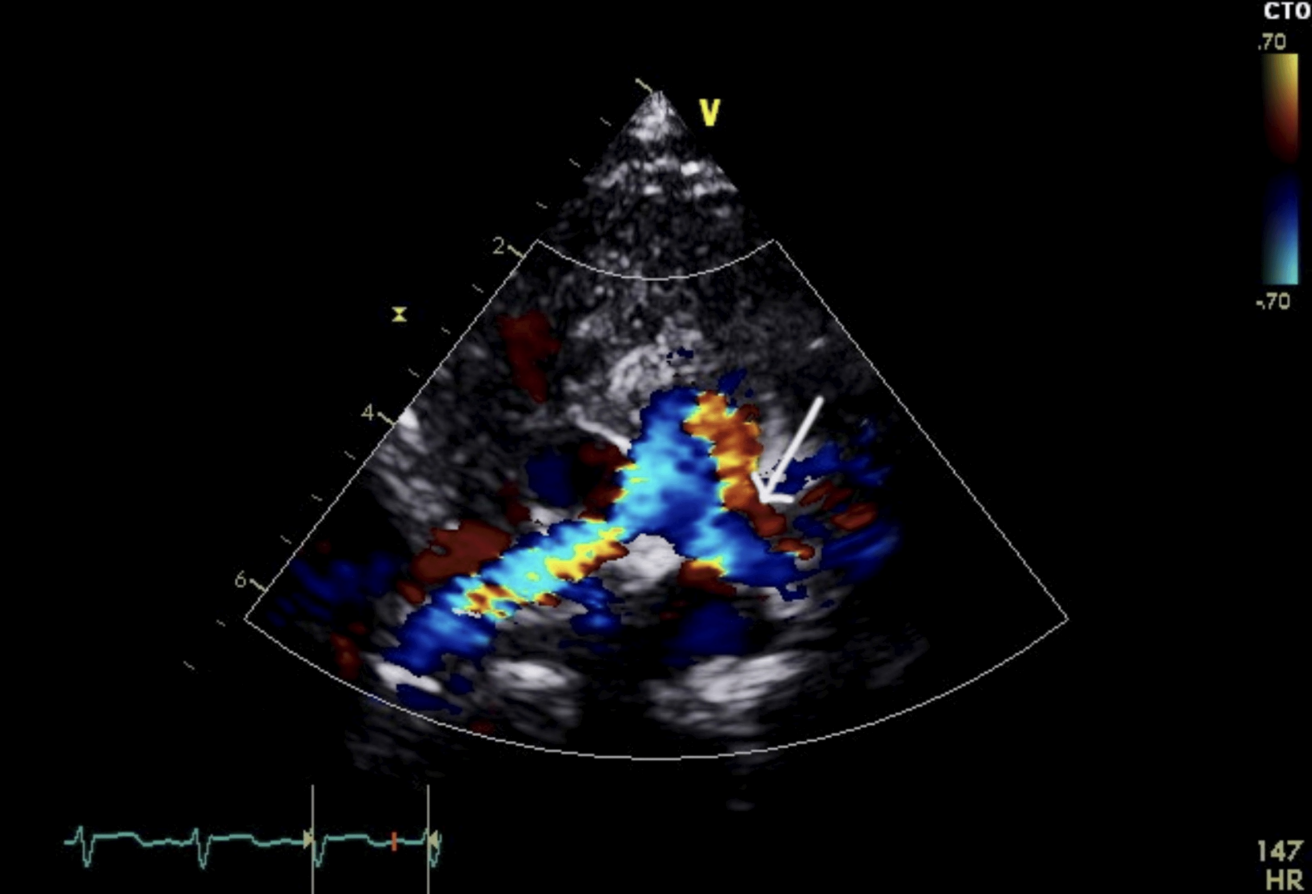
Echocardiogram image of case 1 demonstrating a significant patent ductus arteriosus (PDA) characterized by a bidirectional shunt, predominantly oriented from left to right.
